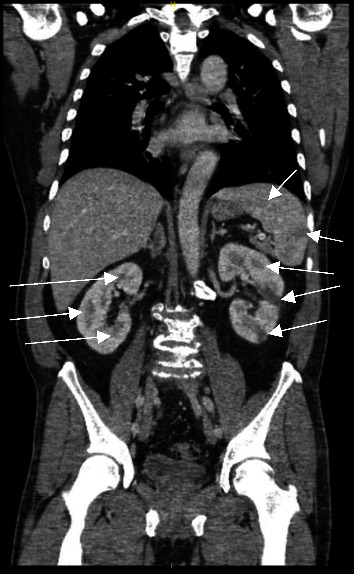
The patient's initial CT scan of the chest, abdomen, and pelvis was remarkable for infarcts in the spleen and bilaterally in the kidneys.
